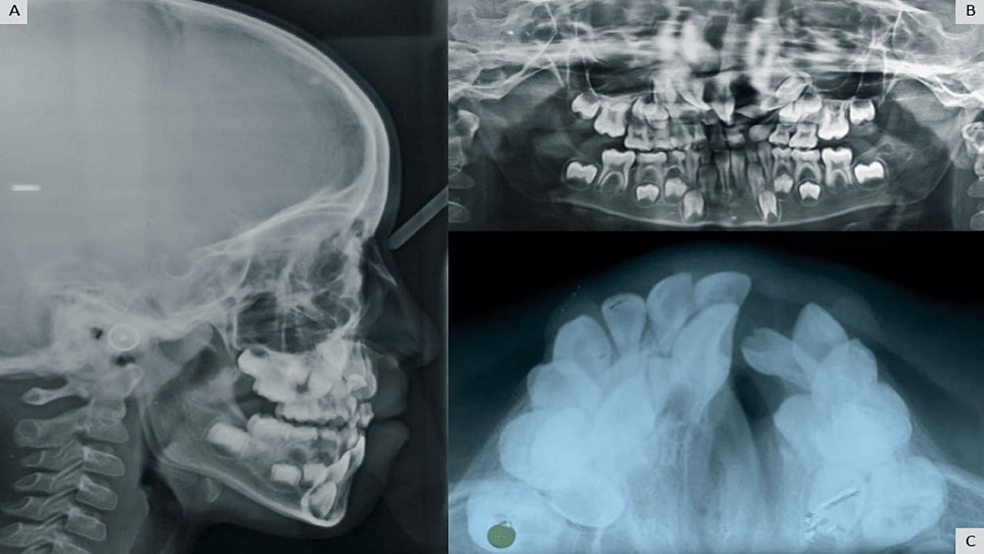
Pre-treatment radiographs: (A) lateral cephalogram, (B) OPG (orthopantomogram), and (C) maxillary occlusal
